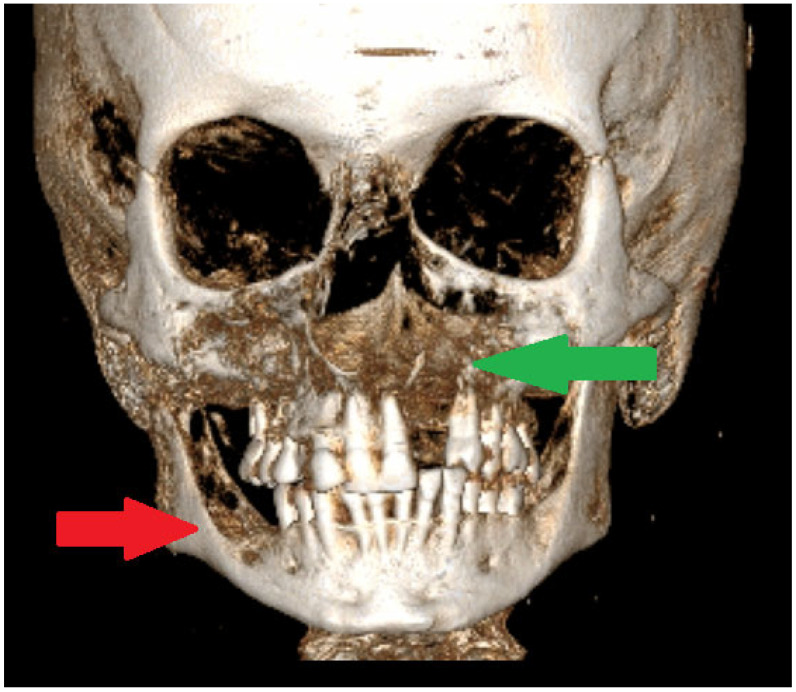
A CBCT-3D of jaw bones can greatly influence the scope of each surgical approach. Smaller bone lesions could be scheduled for a biopsy under local anesthesia. When, additionally, on facial skin, there are discoloured patches suggesting an early BCC (basal cell carcinoma), it is advisable to take two biopsies at the same time to improve the diagnosis of GGS. It is important to remember that GGS can be confirmed based on radiological and pathological evaluation and detailed clinical analysis. The following minor and major criteria for GGS confirmation were established [1,2,3]. The major criteria for NBCCS were as follows: two or more BCCs (basal-cell carcinomas) or one BCC before 20 years, the presence of two or more OKCs (odontogenic keratocysts), at least >3 cutaneous palmar or plantar pits, a 1st degree relative with NBCC (Nevoid basal cell carcinoma syndrome), and the presence of a medulloblastoma. On the other hand, the minor criteria for NBCCS were as follows: the presence of macrocephaly following the height adjustment; the presence of congenital orofacial defects such as frontal bossing, cleft palate, and hypertelorism; the presence of skeletal abnormalities such as syndactyly, pectus deformity or scapula defects, also the occurrence of a radiological abnormality such as fusion of the vertebras; hemivertebras; the bridging of Sella turcica and morphological defects of the hands and feet; the presence of an ovarian fibroma; and signs of bifid or fused ribs [1,2,3]. In many cases, during oral cavity, head, and neck evaluations, it is quite easy to see multiple cystic bone lesions, the presence of facial skin lesions/BCC, the presence of palatal defects and anomalies, and frontal bossing. In CBCT, it is easy to see a calcified falx cerebri and some disturbances with a high palate. The scope of each lesion might have different manifestations. In jaw surgery, special attention should be focused on extensive, expansile bone lesions causing visible bone disfigurement and bone asymmetry, and rarely occurring in the mandibular condyle head and temporomandibular area (TMJ). When TMJ structures are affected and the destruction of the joint occurs, some accompanying syndromes like joint pain, the inability to open the mouth, LMO-limited mouth opening, swelling and inflammation near the TMJ area, and problems with biting and chewing (red arrow). In elderly patients, this might also cause other problems related to prosthesis usage, overloading of the opposite side healthy joint, the growth of severe pain levels, and inflammation-related changes in the TMJ, which is where the OKC might spread [4,5]. Secondly, the problem is quite serious when a unicystic lesion of OKC has a polycystic appearance without borders and an expansive character causing bone asymmetry and cortical thickening, especially when present in the maxillary sinus, anterior maxillary bone, and alveolar process of the maxillary bone, or if any spread towards the nasal cavity (green arrow). Many approaches can be used to reduce the scope of resection, but the authors promote bone estectomy and allograft bone usage to strengthen the bones.
